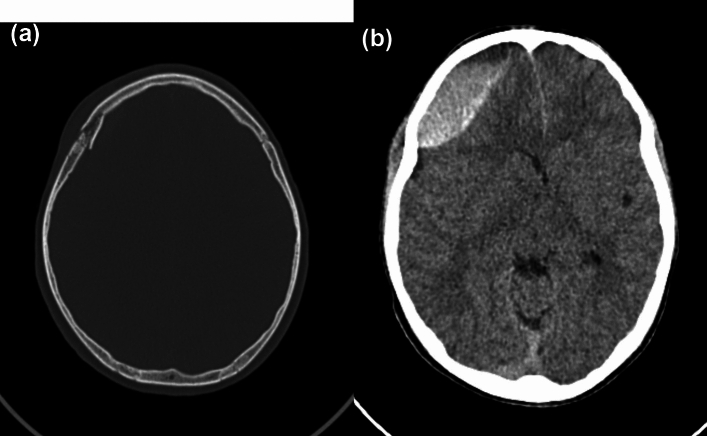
A 12-year-old player presented with a Glasgow coma scale (GCS) of 13/15. Axial CT demonstrated a comminuted, depressed fracture of the right fronto-parietal bone (a) with a large underlying extradural haematoma causing mass effect with 5 mm of midline shift to the left and subfalcine herniation (b)
